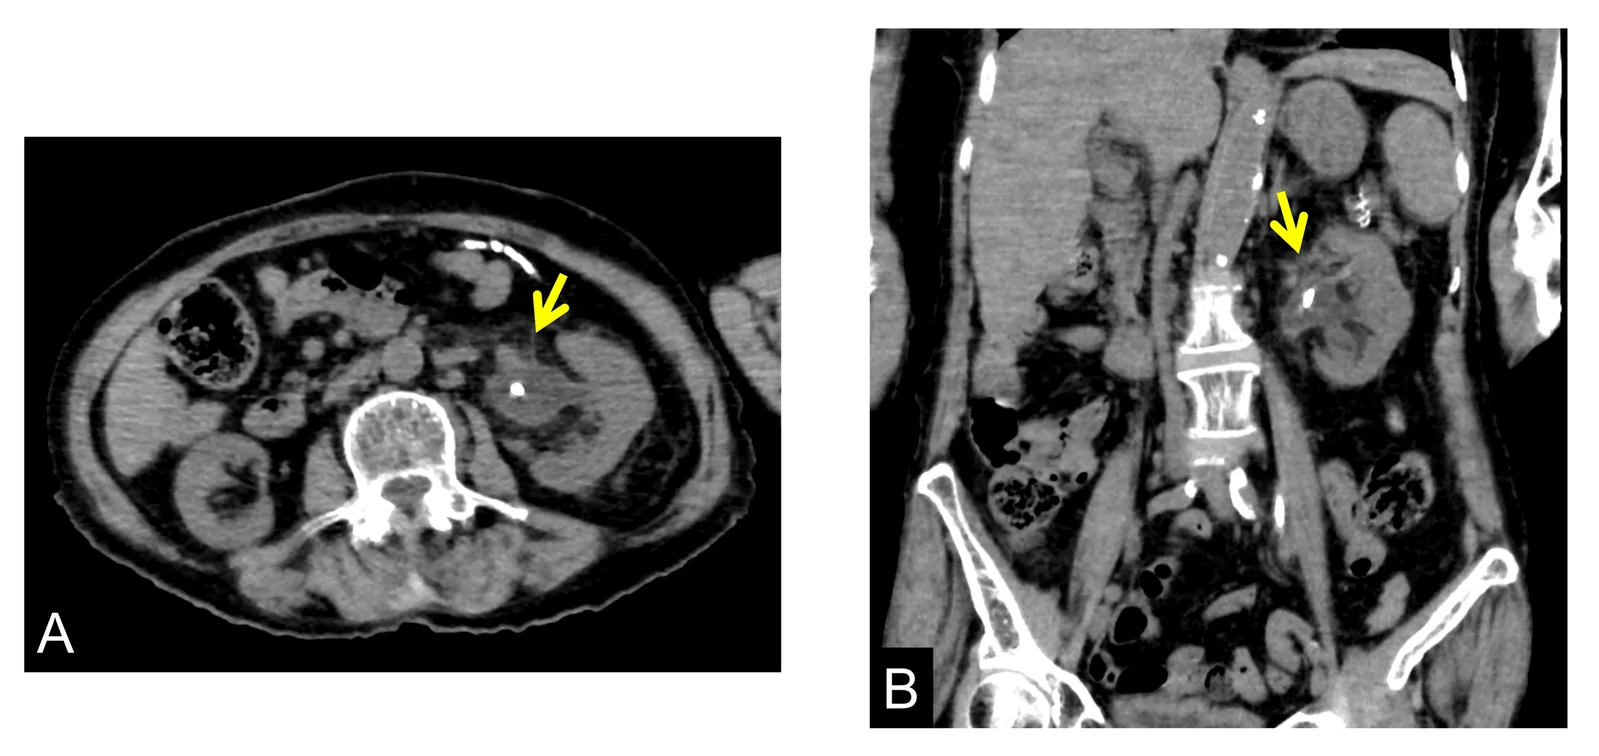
Computed tomography demonstrating left hydronephrosis despite an indwelling ureteral stent. A) Axial computed tomography image showing left hydronephrosis despite the presence of a ureteral stent. The yellow arrow indicates the dilated left renal pelvis. B) Coronal computed tomography image demonstrating left hydronephrosis and the indwelling ureteral stent. The yellow arrow indicates left hydronephrosis.
